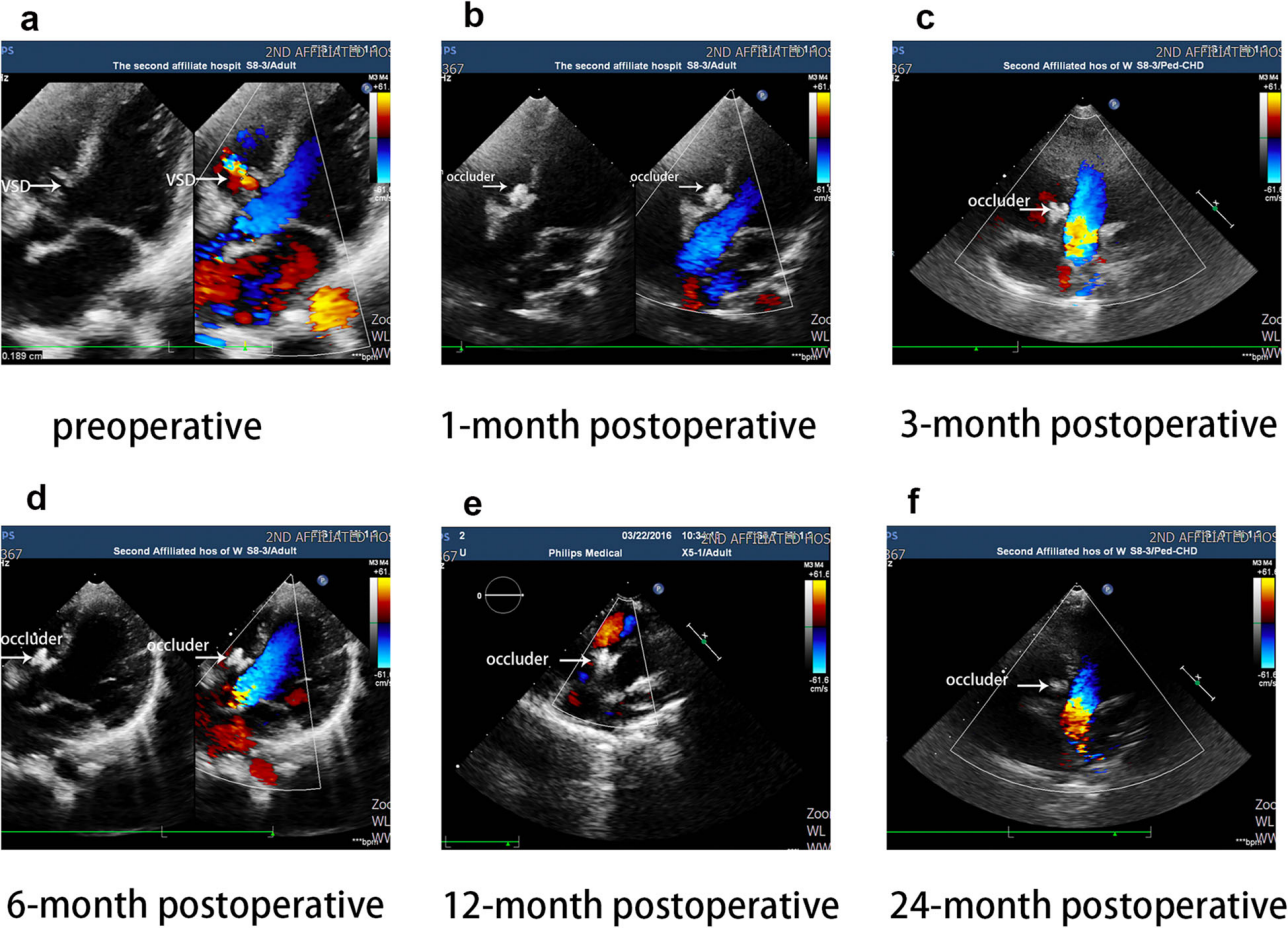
Echocardiography: four-chamher view with VSD, from preoperative to 2 years follow-up (26-month-old patient). VSD:ventricular septal defect; occluder: the device closed the defect without residual shunt. a: preoperative(d = 3.5 mm); b: at 1 month after the procedure; c: at 3 months after the procedure; d: at 6 months after the procedure; e: at 1 year after the procedure; f: at 2 years after the procedure
